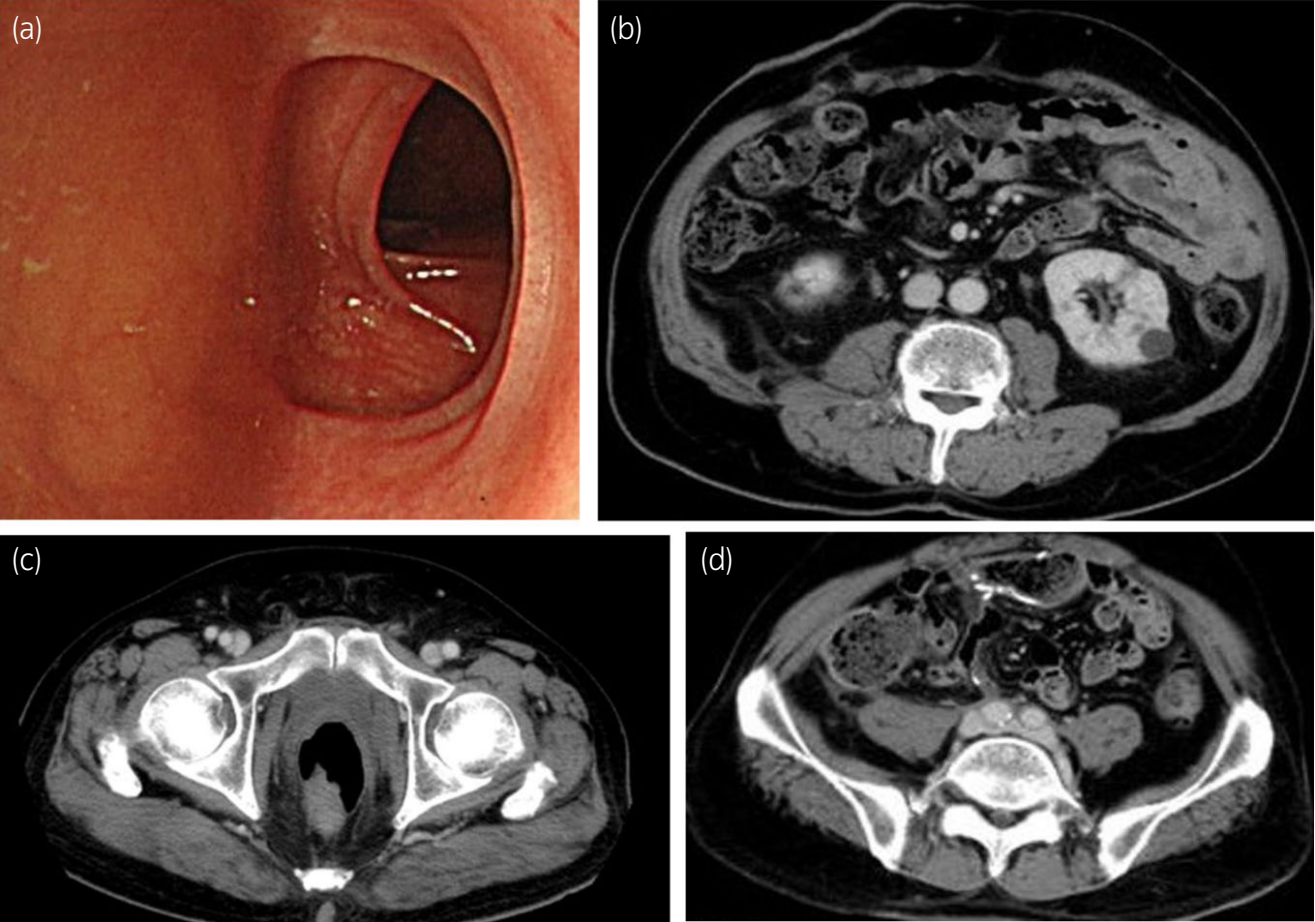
Complete response after dd‐MVAC treatment. Colonoscopic findings in the descending colon (a) and CT scan findings (b–d).
